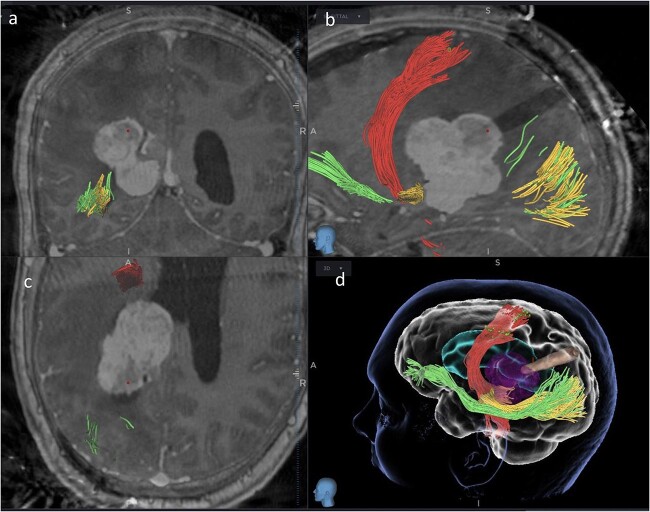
Coronal (a), sagittal (b) and axial (c) views of the merged images from intra-operative CT scans (O-Arm) with pre-operative MRI tractography. The tubular retractor is in situ. (d) Virtual 3D reconstruction of the tubular retractor (brown) in the merged images and its relationship with the tumour (purple), the CST (red), the IFOF (green), and the OR (yellow).
